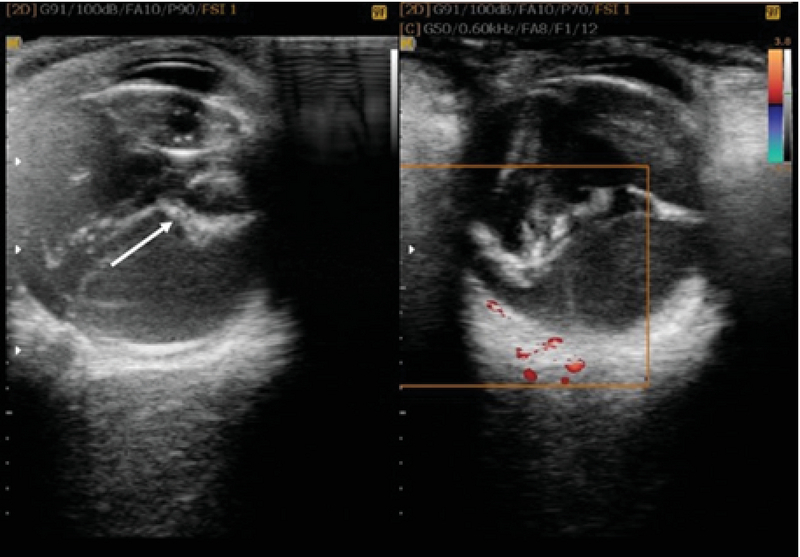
Color Doppler ultrasonography shows no flow in the membrane seen in the left figure, ruling-out RD. The avascular membrane has been marked in the right figure.
